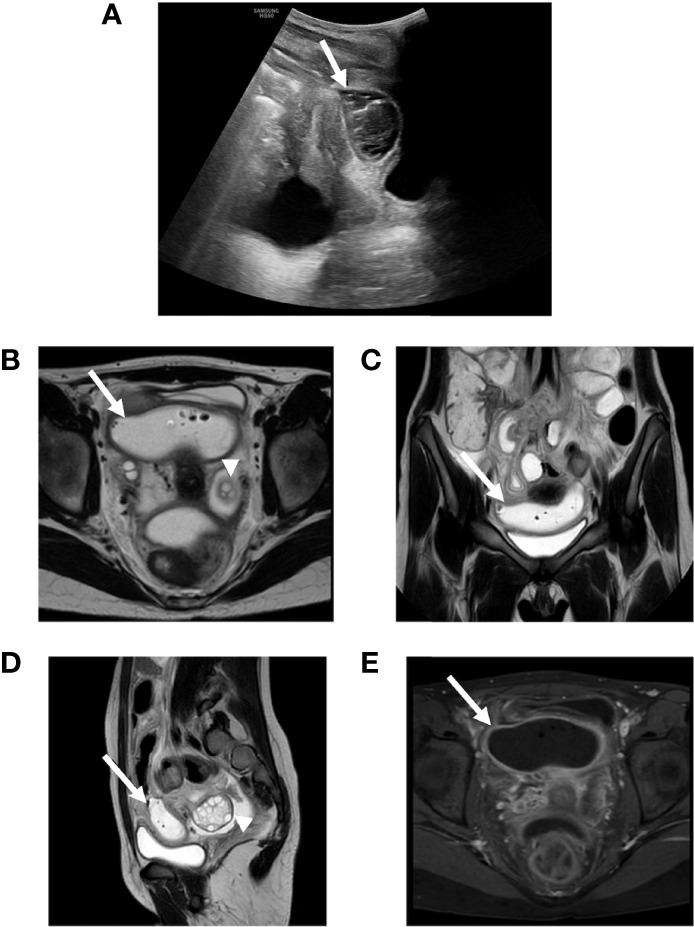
(A) Abdomen ultrasound showing an encapsulated abscess between bladder and uterus (arrow). (B–E) Abdomen MRI showing a pelvic large fluid-filled abscess (arrows) with air bubbles such as for bowel perforation (B–D: T2 weighted) with parietal contrast enhancement (E: T1 weighted) and normal adnexal region (arrowheads).
